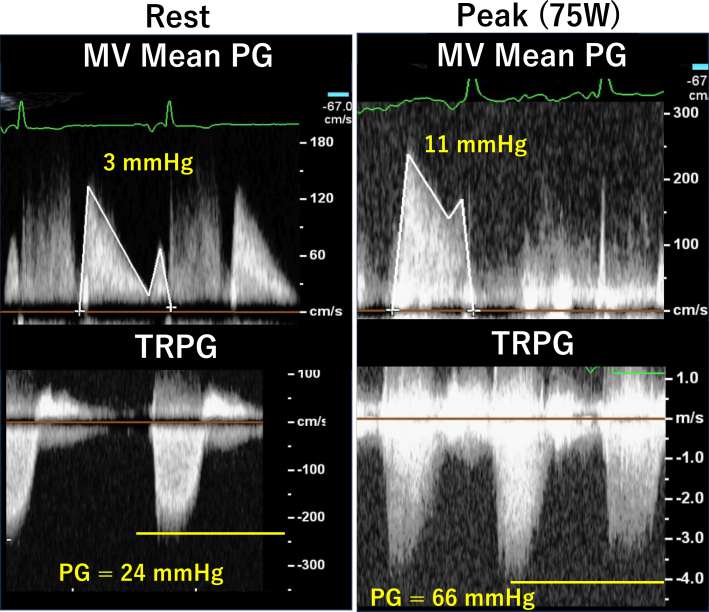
Exercise Stress Echocardiography Findings The MV mean PG was elevated from 3 to 11 mm Hg without worsening of mitral regurgitation. The TRPG was also elevated from 24 mm Hg to 60 mm Hg at a 75-W load on the ergometer. MV = mitral valve; PG = pressure gradient; TRPG = tricuspid regurgitation pressure gradient.
